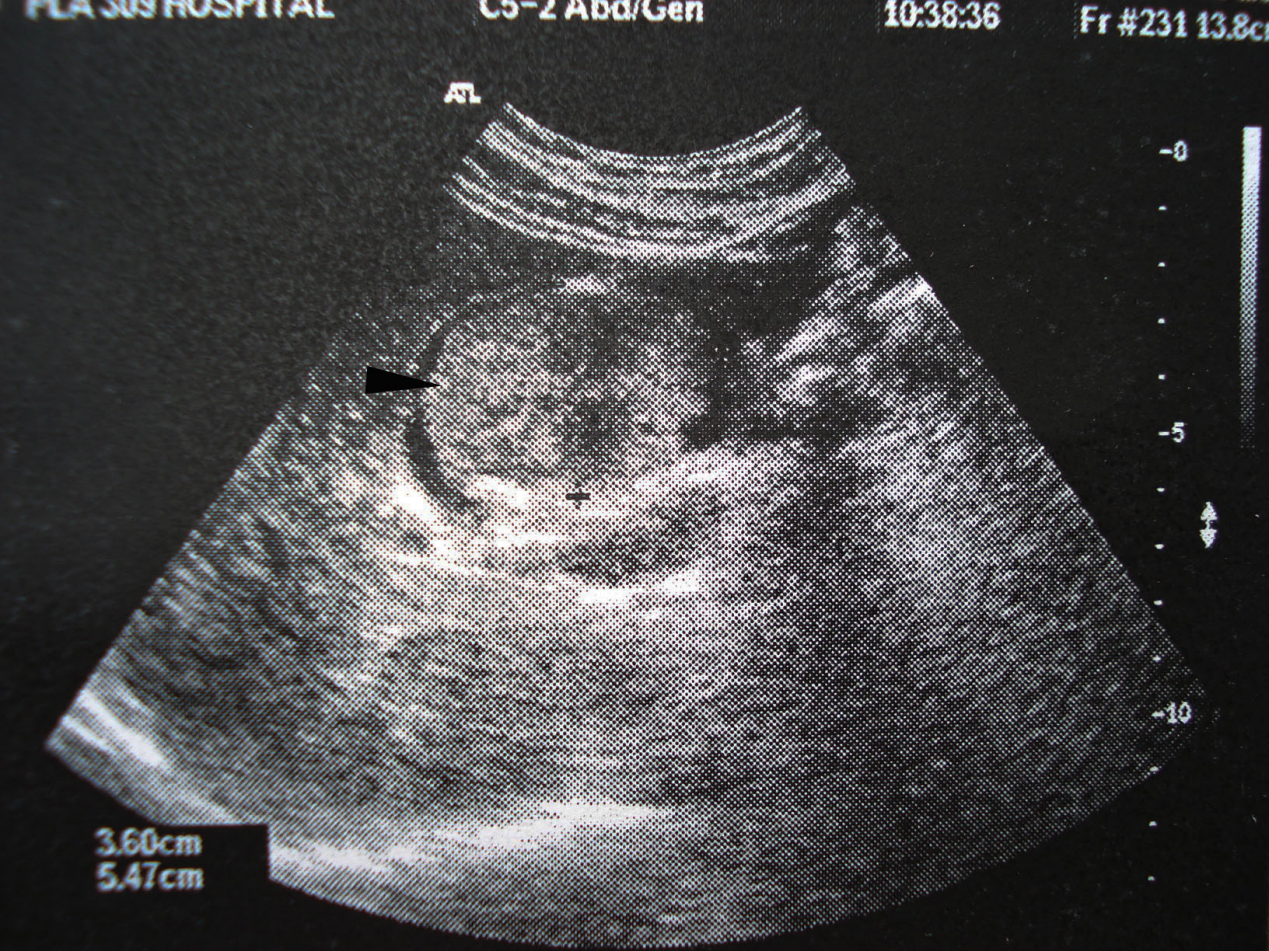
Abdominal ultrasound examination showing a mass (arrow) in the gallbladder.
